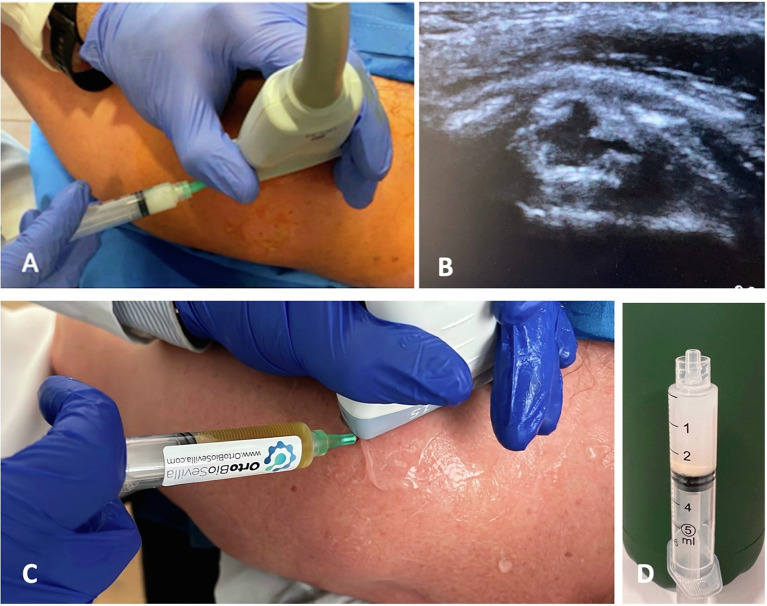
(A) Calcium aspiration in a syringe. (B) Ultrasound image of the sign of Nidus (break in the thickness of the supraspinatus (long axis). (C) Infiltration with intralesional PRP (3mL in nidus) and 6 mL in the bursa. (D) An image showing a syringe with the calcium deposits after the barbotage technique.
